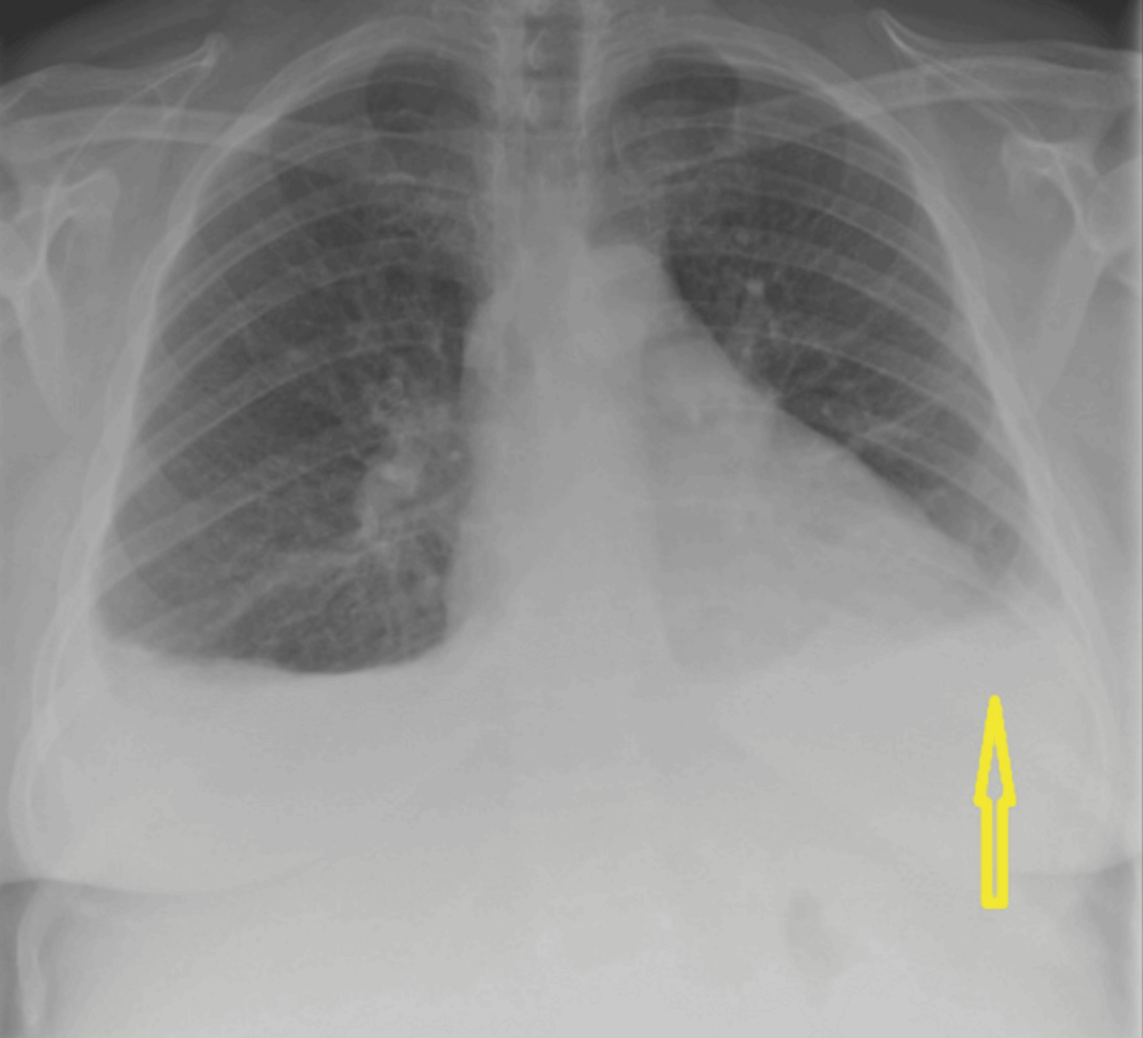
Chest X-ray showing pleural effusion (yellow arrow)
